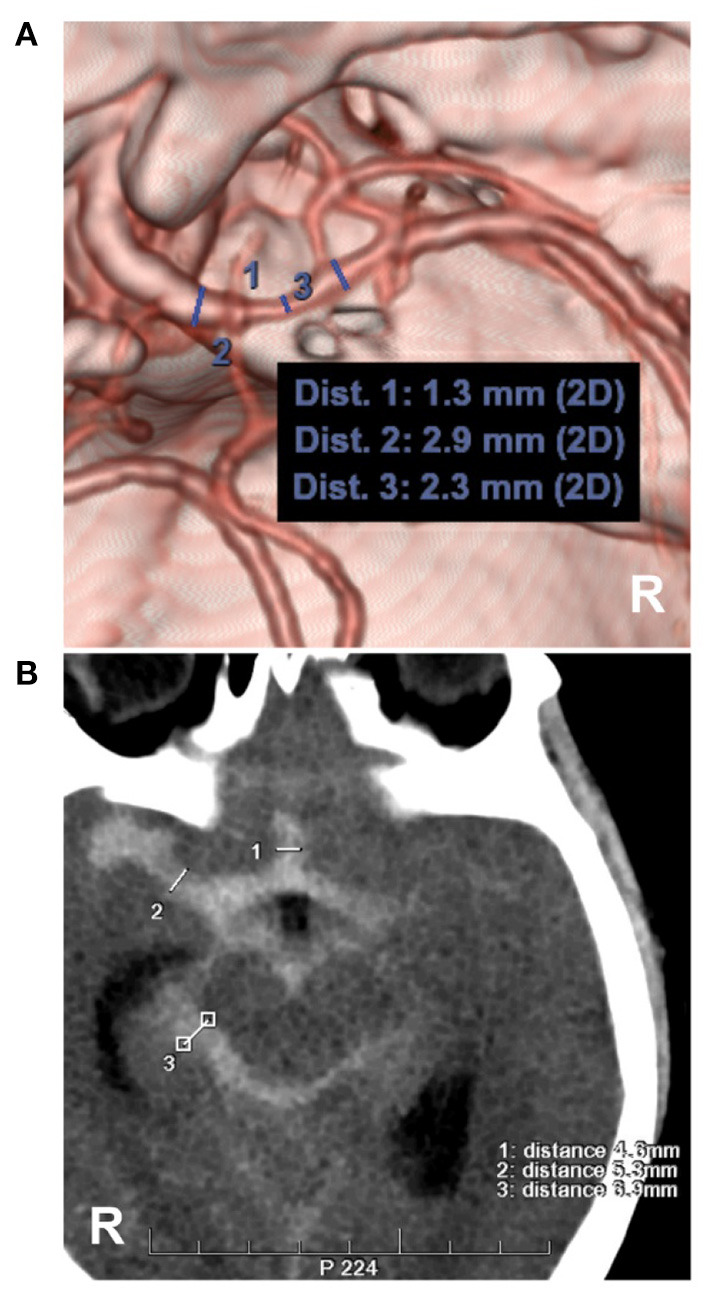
Measurements of intracranial vasospasm and SAH thickness. (A) CTA showing the diameter measurement of the MCA. No. 1, 2 and 3 showing the diameters of the different locations of the MCA, the location of No. 1 had a moderate vasospasm (33–66%, 1.3/2.3 and 1.3/2.9). (B) CT showing the measurements of the Barrow Neurological Institute scale, No. 1, 2 and 3 showing the subarachnoid hemorrhage thickness (4.6, 5.3, 6.9 mm), by the measurement across the thickest-appearing regions of the cistern or fissure. CT, computed tomography; CTA, computed tomography angiography; MCA, middle cerebral artery; R, right.
